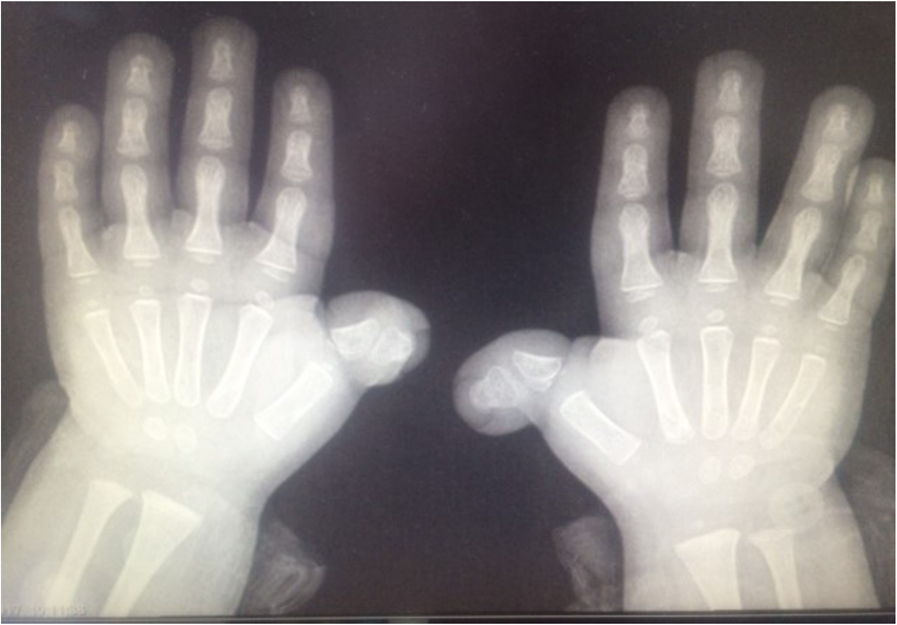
X-rays of the hands. The angulated thumbs are caused by a delta-phalanx
